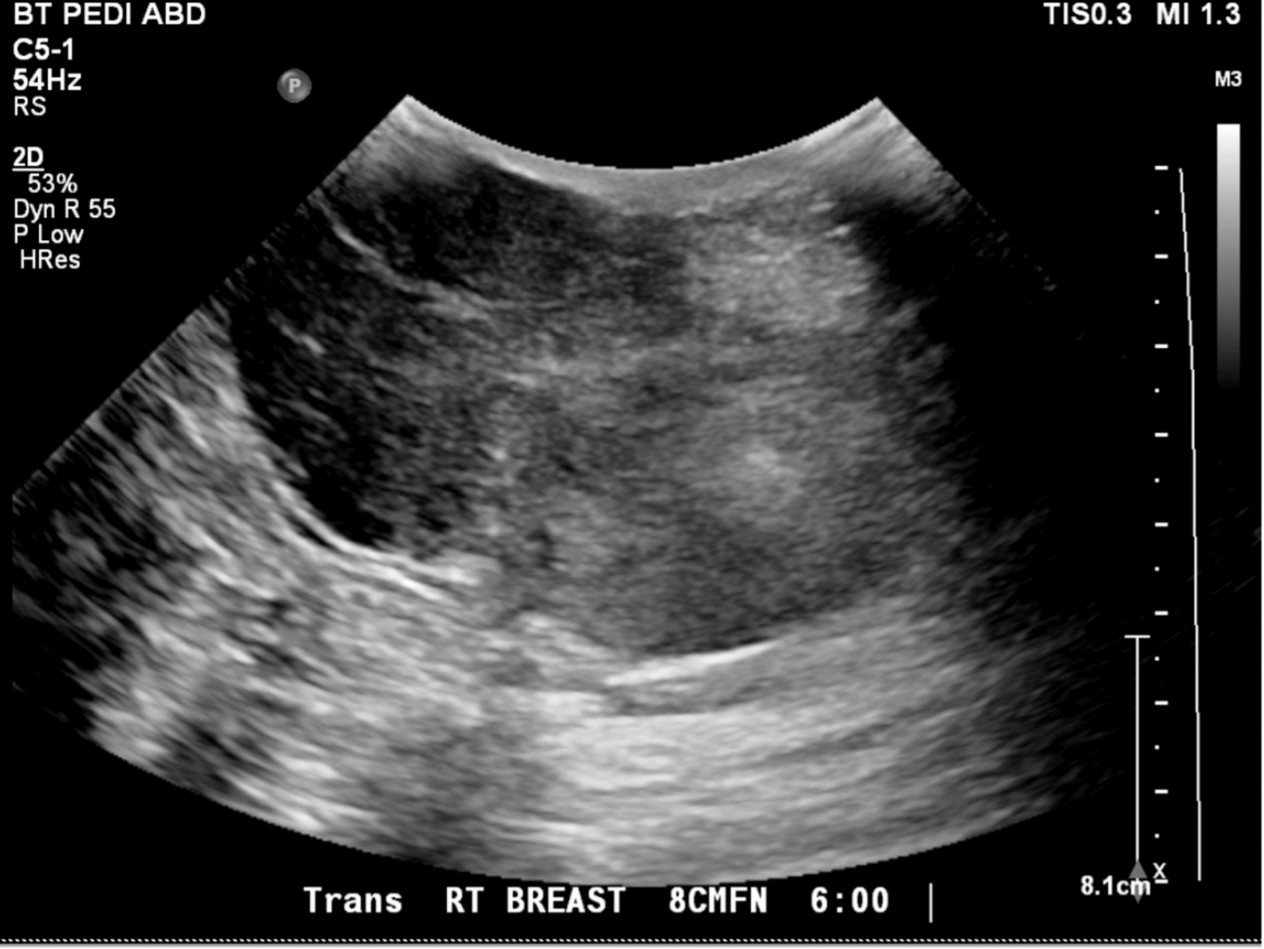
Breast ultrasound image (I) The image is showing a 10.1 x 5.4 x 7.5 cm partially solid mass with concern for possible malignancy.
